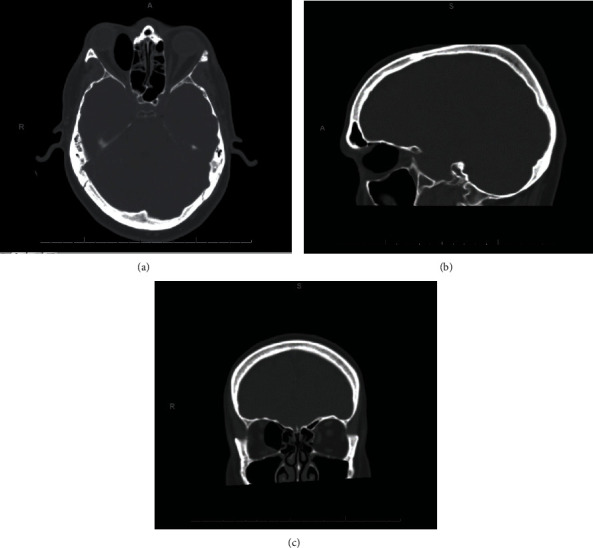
CT scan of patient with (a) axial, (b) sagittal, and (c) coronal views showing a 36 × 26 × 16 mm collection of gas in the medial aspect of right orbit causing displacement of intraorbital structures and resultant.
